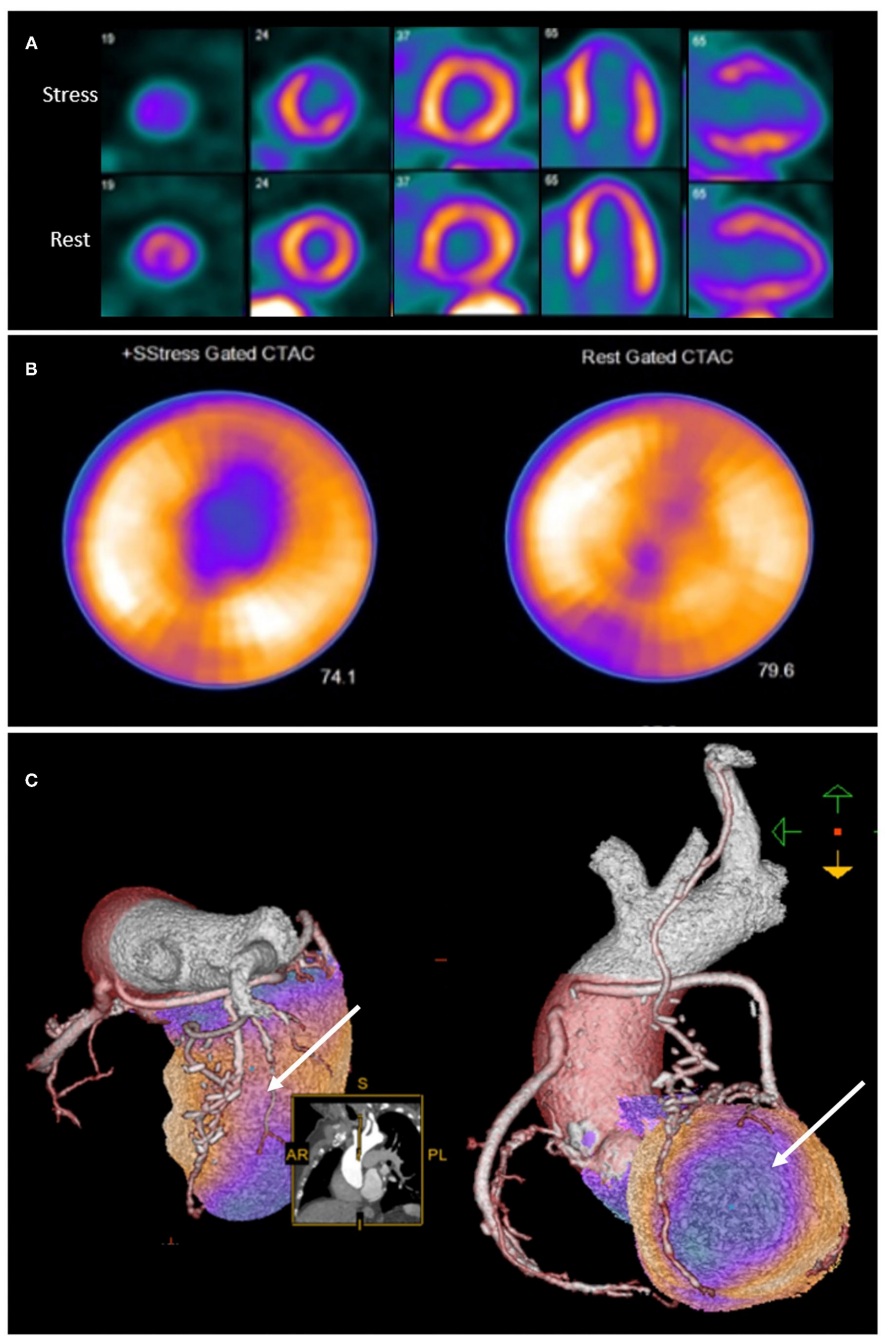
Rubidium-82 PET-CT with adenosine stress in an 86-year-old male with previous coronary artery bypass grafting. PET-CT images (A,B) obtained at stress and rest demonstrate a reversible perfusion defect in the mid to apical anterior segments extending into the apex. Cardiac hybrid imaging with three-dimensional fusion of PET-CT with CT coronary angiography enables localization of ischaemia to a coronary artery territory (C). CT coronary angiography reveals a patent LIMA to LAD graft with good distal opacification, and obstructive plaques in the proximal and mid segments of an intermediate artery (white arrow), responsible for the reversible perfusion defect demonstrated.
